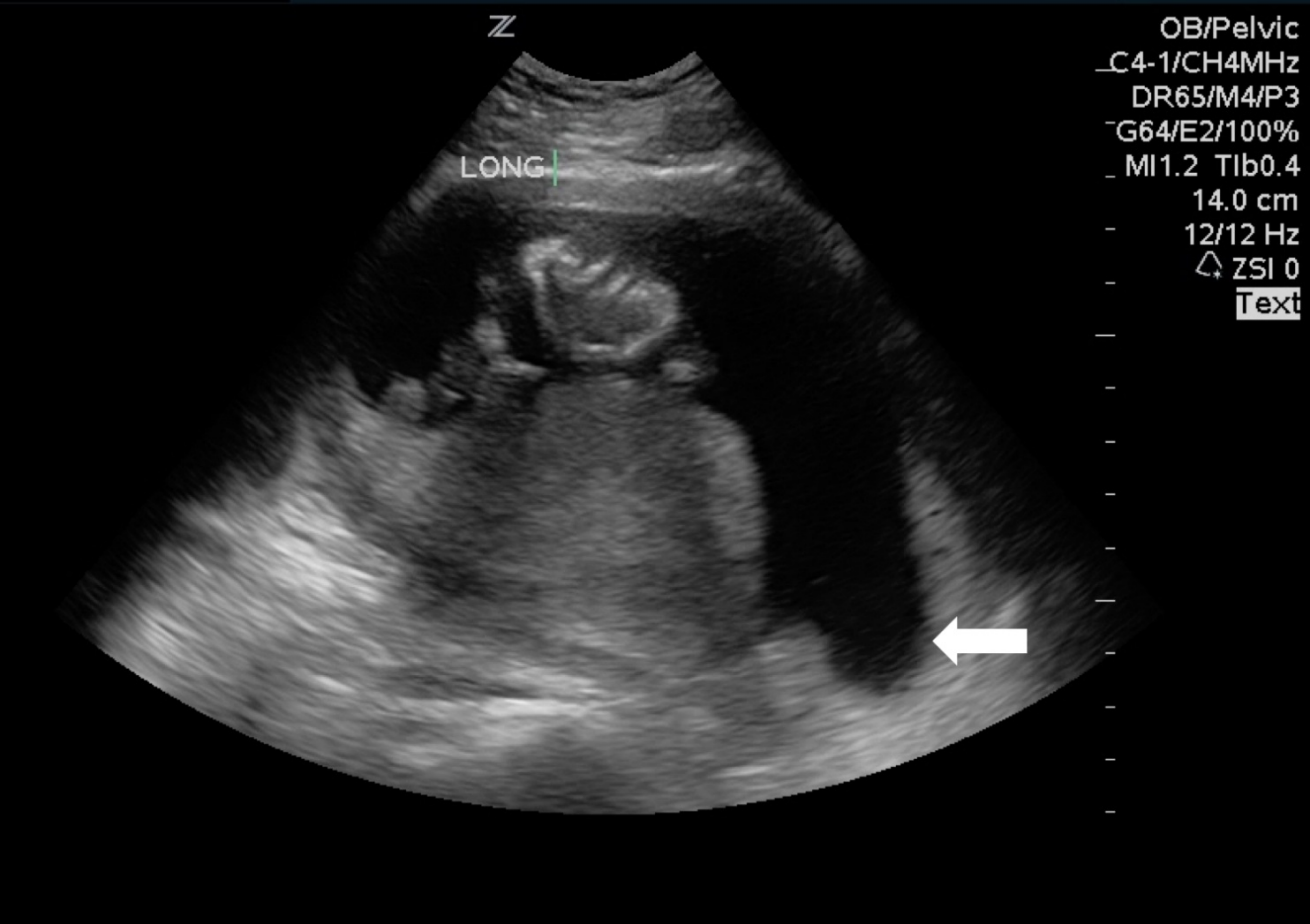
Sagittal imaging of a gravid uterus. Point of care sonographic image of uterus with cervical funneling (white arrow).
